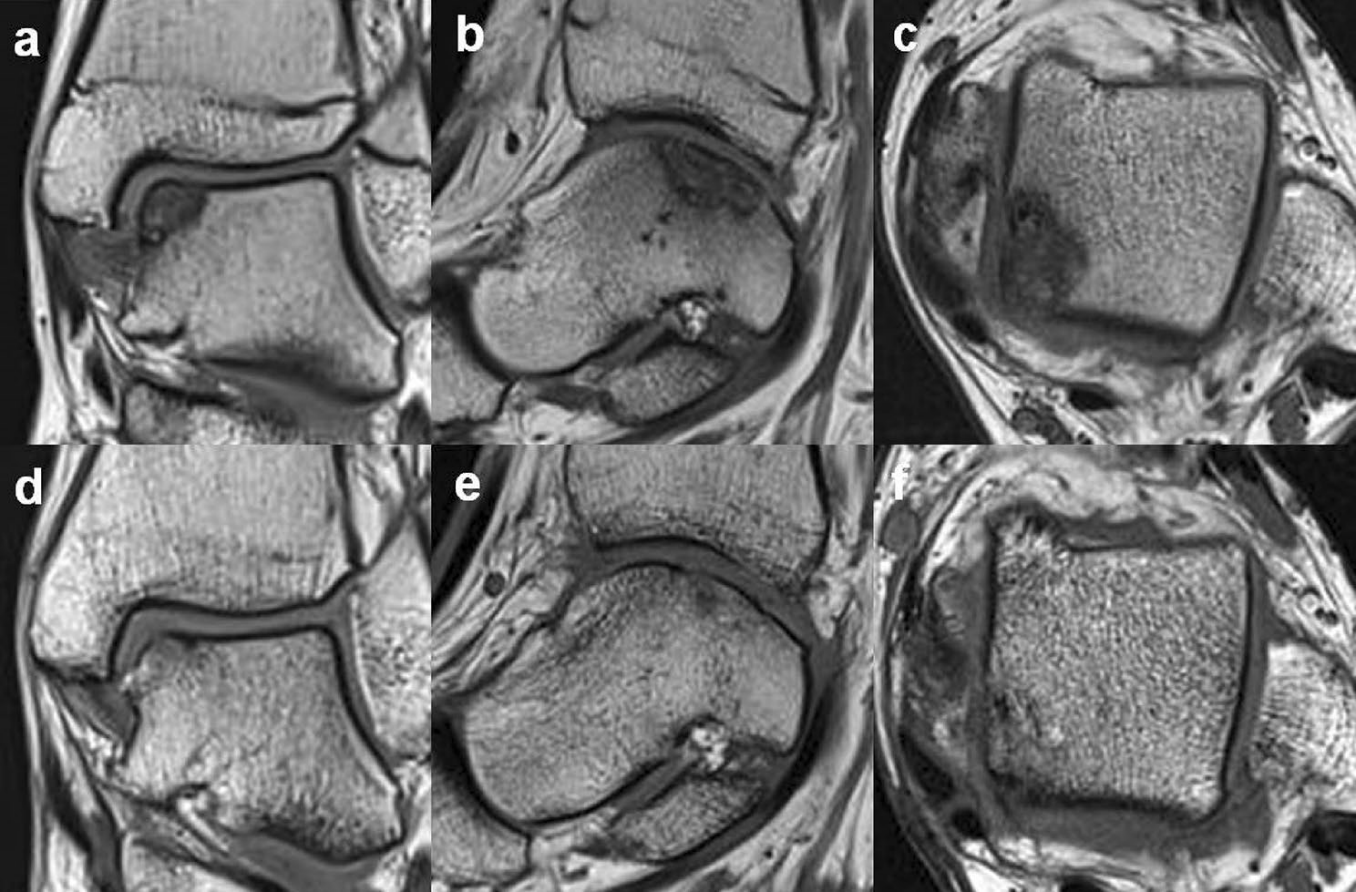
Preoperative T1-weighted MRI scans of a 15-year-old male patient show low signal intensities of the osteochondral fragment on (a) coronal, (b) sagittal, and (c) axial images. The fragment appeared non-viable for bone healing. (d), (e), (f) On the 24-month postoperative MRIs, the signal intensities increased to levels similar to the underlying talus.
